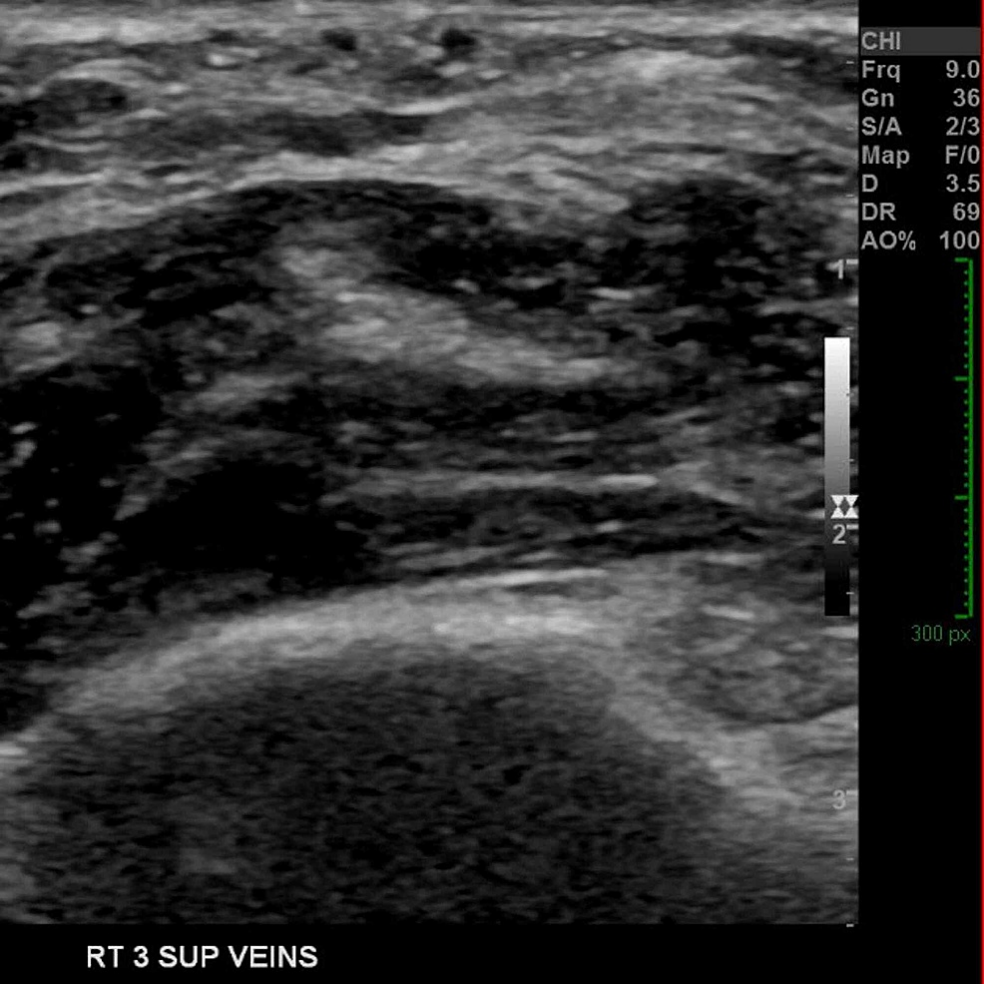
Right antecubital fossa ultrasound scan showing three linear cord-like bands (1 mm each) lying laterally within the antecubital fossa, and immediately underneath the skin.
